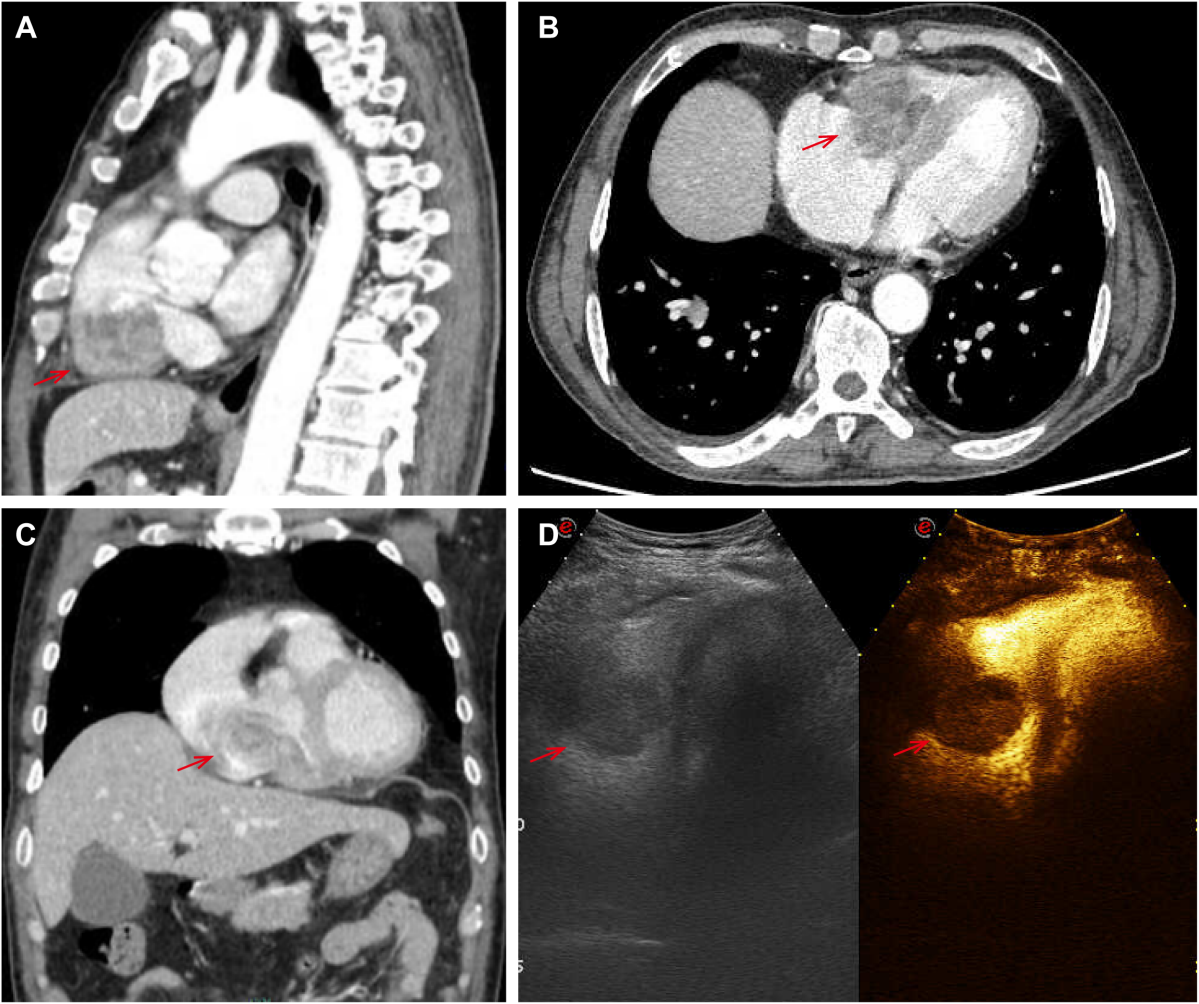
Pictures (A–C) showed cardiac metastasis on the right ventricle at chest-enhanced CT scans; the echocardiogram showed a hypoechoic mass of 5.3*3.2cm in the right ventricle on picture D.
